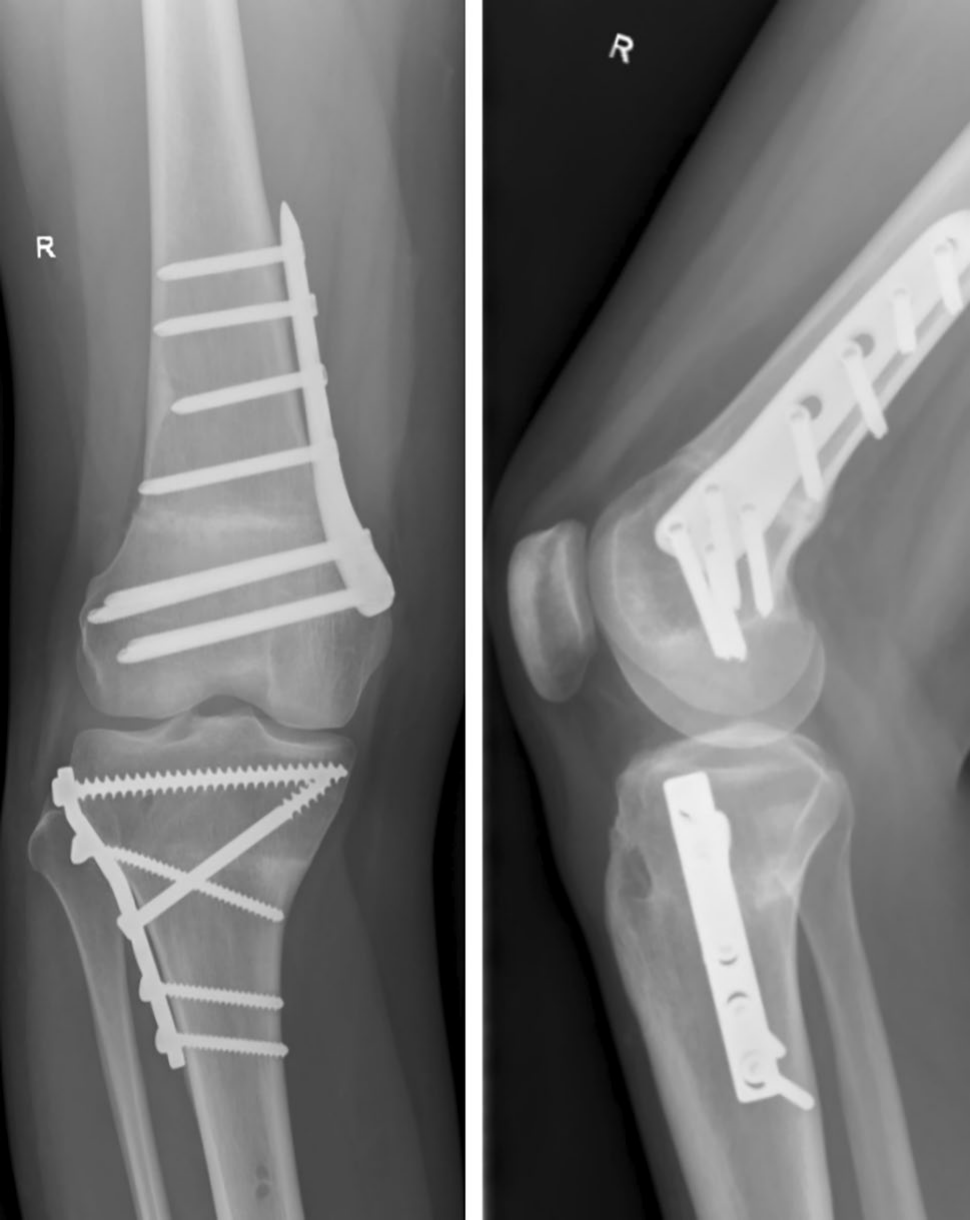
Radiography of the knee in two planes of a 21 year-old woman with severe ‘inwardly pointing knee syndrome’ six months after double-level (12°-femoral external and 12°-tibial internal rotational) osteotomy and osteosynthesis (with 5-hole DCP plate tibial and MDF TomoFix femoral), bony consolidation
